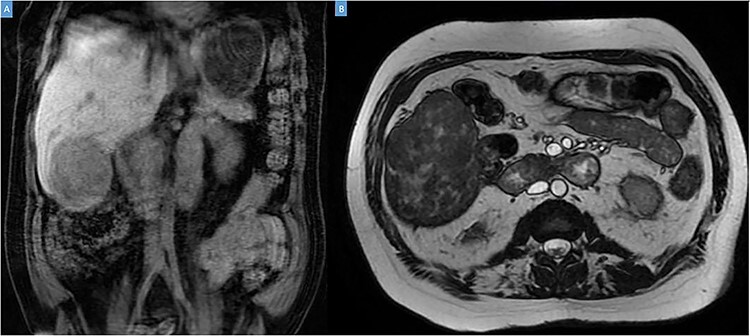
Coronal (A) and axial (B) sections of MRI showing the peripheral liver tumor.
